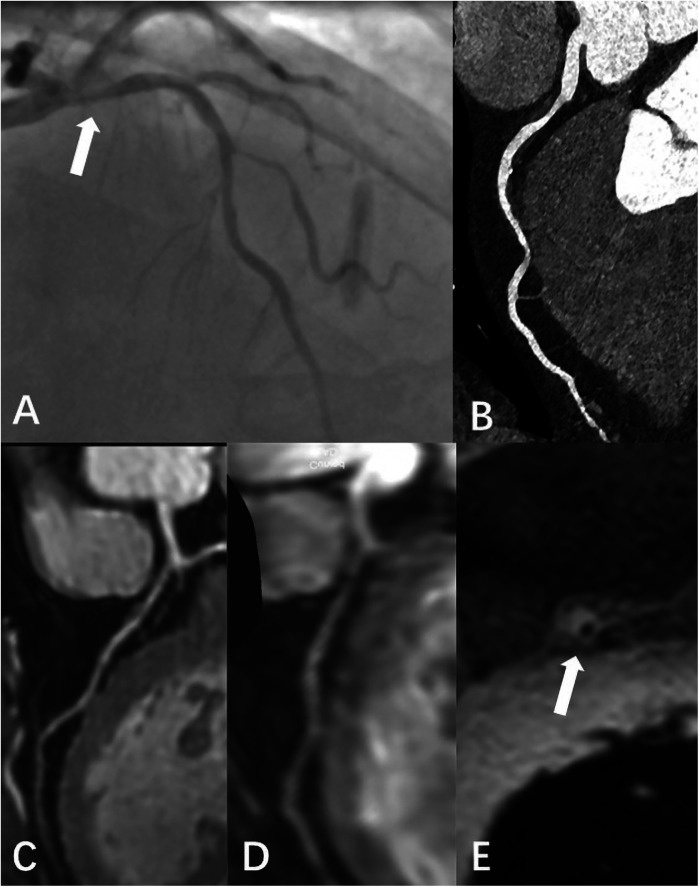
Male, 59 Y, with chest pain. (A) Catheter-based x-ray coronary angiography indicated a 70% stenosis at the origin of the LAD. (B) CTA revealed non-calcified plaque surrounding the lumen at the proximal LAD, with moderate stenosis. (C) 3D BTFE showed a localized signal reduction in the proximal vessel wall. (D) MSG-EPI indicated moderate stenosis in the proximal lumen. (E) Axial T1-weighted fat-suppressed black-blood sequence suggested the presence of a high-signal plaque surrounding the vessel wall.
